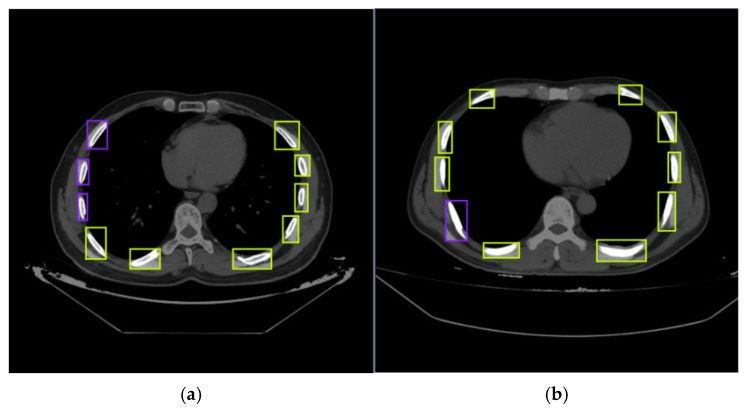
CT images labeled according to fractured and non-fractured areas: Green boxes indicate non-fractured regions, and purple boxes indicate fractured regions: (a) random sample 1 from the dataset; (b) random sample 2 from the dataset.
